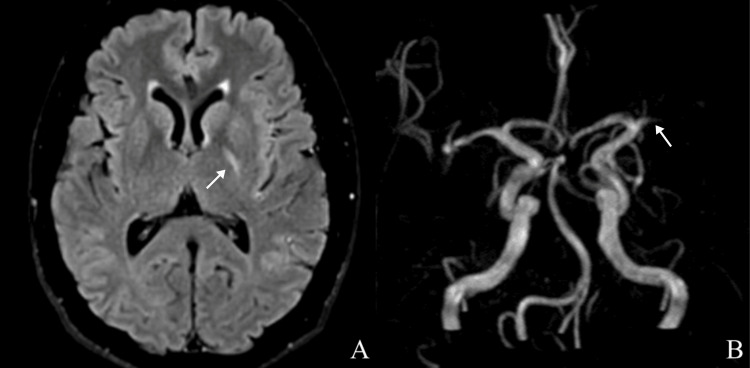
A) T2-FLAIR MRI demonstrating a hyperintense lesion localized in the posterior limb of the left internal capsule (arrow indicates lesion site). B) Angio-MRI with a 3D reconstruction of the cerebral vasculature, demonstrating a vascular occlusion at the left middle cerebral artery (arrow points to the site of neurovascular occlusion).
